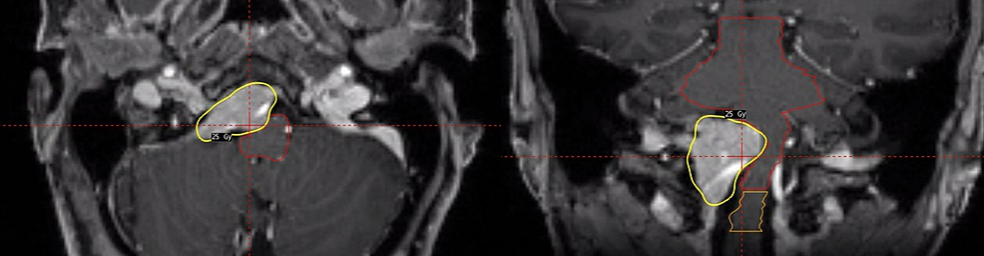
Axial (left) and coronal (right) T1 post-contrast planning MRI of a skull-based WHO grade I meningioma that was treated with 25 Gy in five fractions on the GK Icon. MRI = magnetic resonance imaging; WHO = World Health Organization; GK = Gamma Knife
